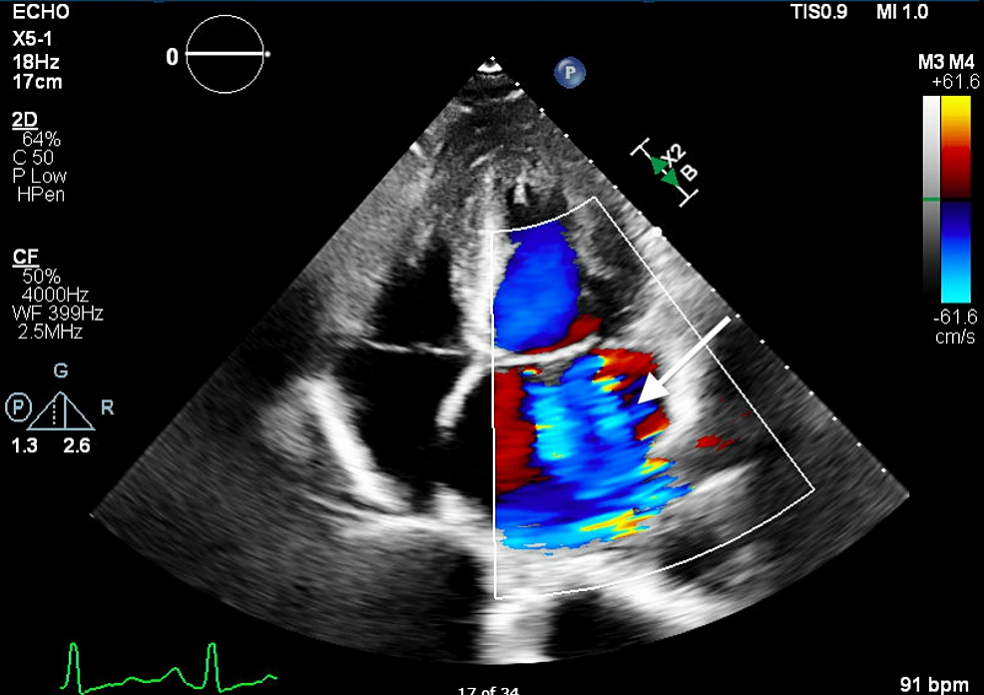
Transthoracic echocardiogram four-chamber view revealing severe torrential mitral regurgitation
